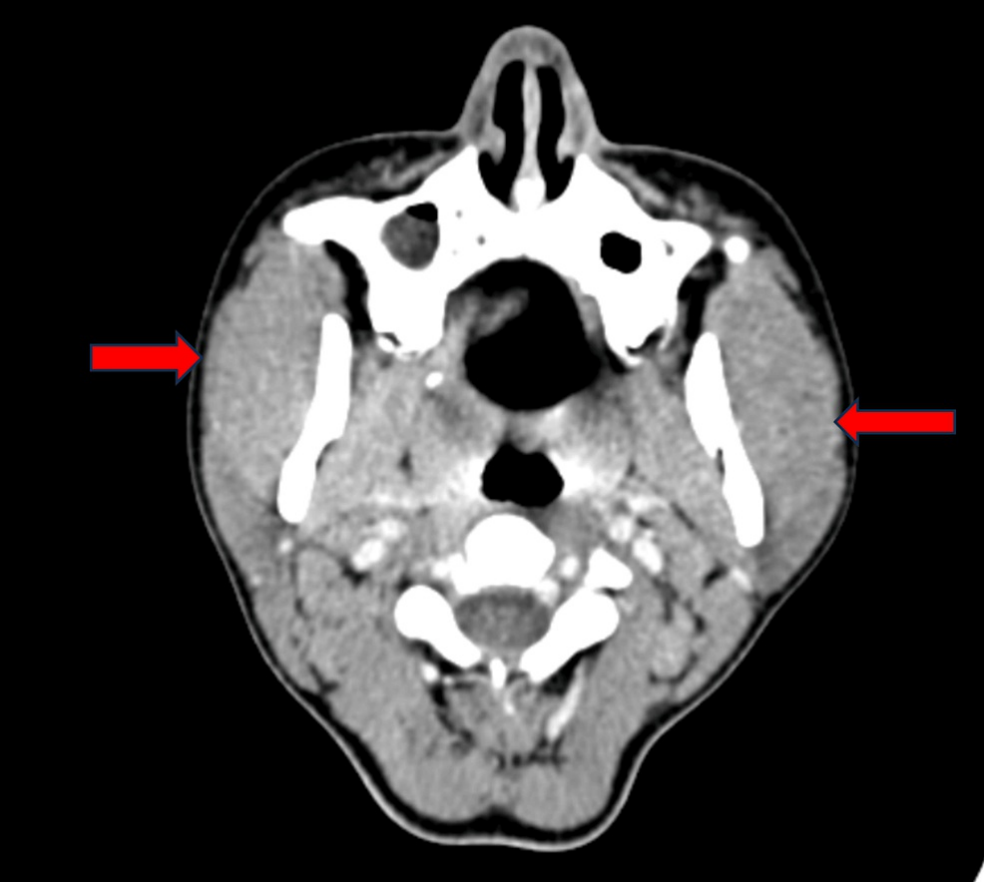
CT scan axial view showing bulky masseter muscle hypertrophy (red arrows)
